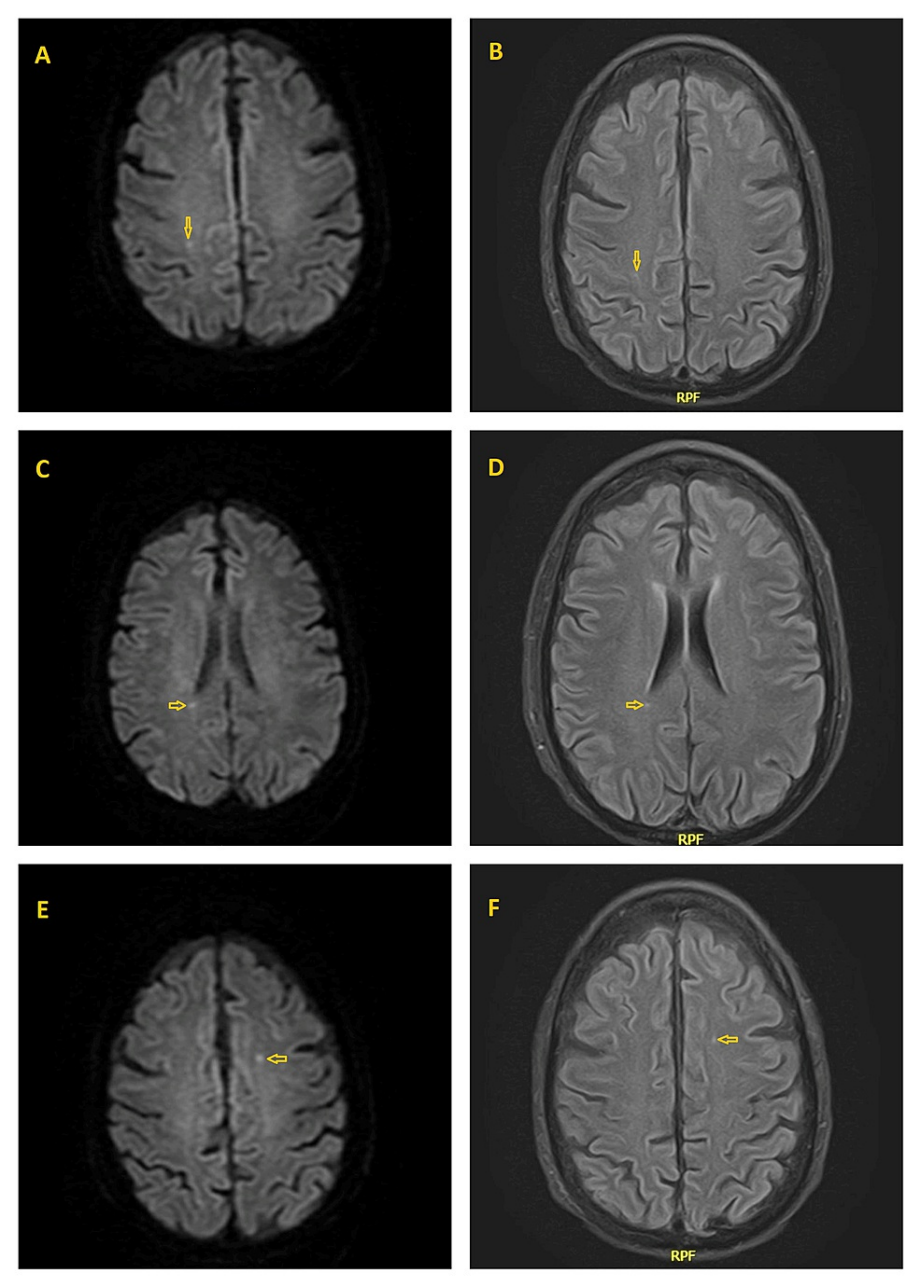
Magnetic resonance imaging (MRI) of the brain Diffusion-weighted MRI (4A, 4C, 4E) and fluid-attenuated inversion recovery (FLAIR) MRI (4B, 4D, 4F) of the brain showing subacute lacunar stroke (yellow arrows) with distribution suggestive of embolic phenomena.
